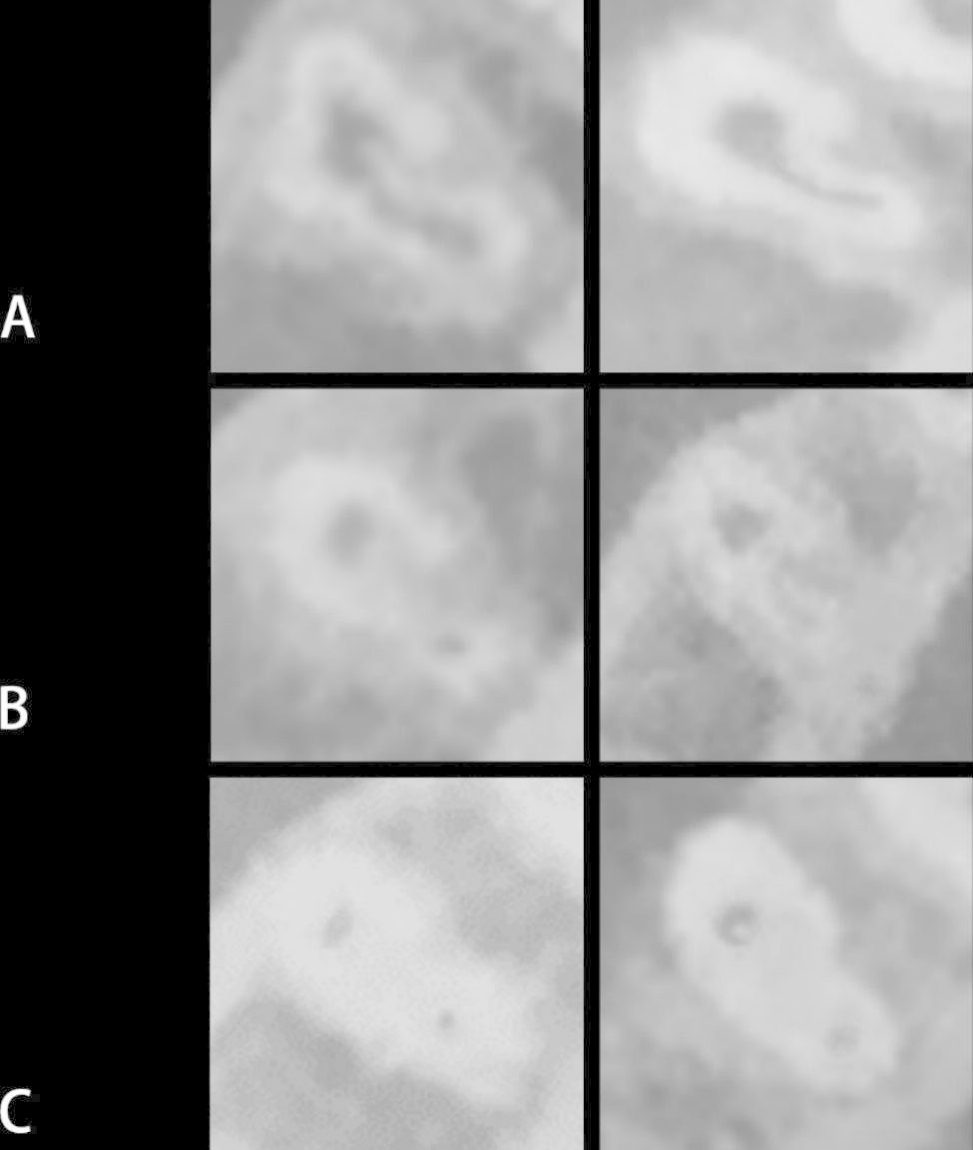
Representative CBCT images of C-shaped root canal in mandibular first premolars (A) C1; (B) C2; (C) C3
